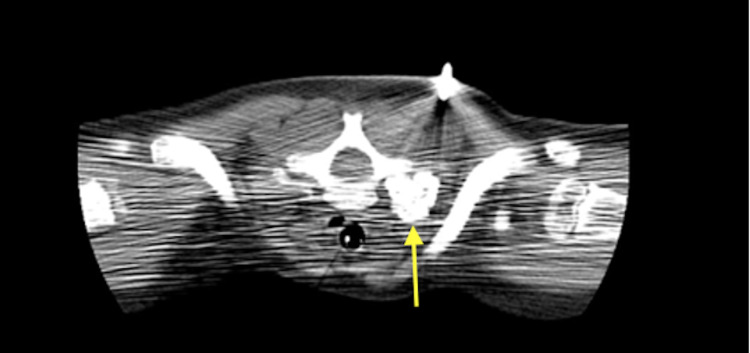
CT-guided bone biopsy of right cervicothoracic paraspinal mass (at yellow arrowhead) that revealed osteochondroma Patient was in a prone position for the procedure. CT - Computerized Tomography
